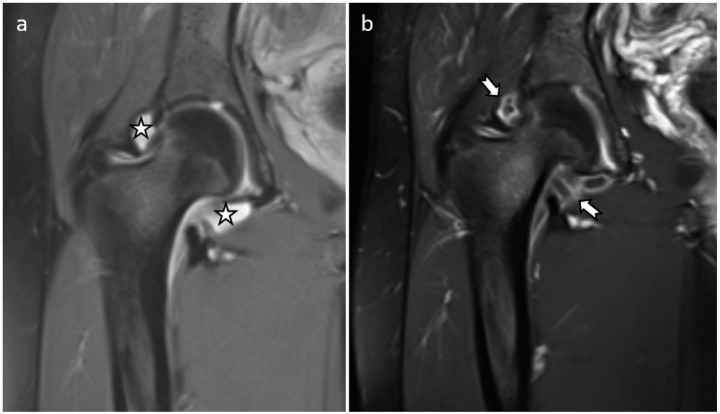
Joint effusion and synovitis. (a) T2-weighted image with fat suppression; (b) T1-weighted image with fat suppression and contrast enhancement. Marked effusion within the right hip joint cavity (asterisk), and marked thickening and enhancement of the synovium (tail-of-swallowtail arrow).
